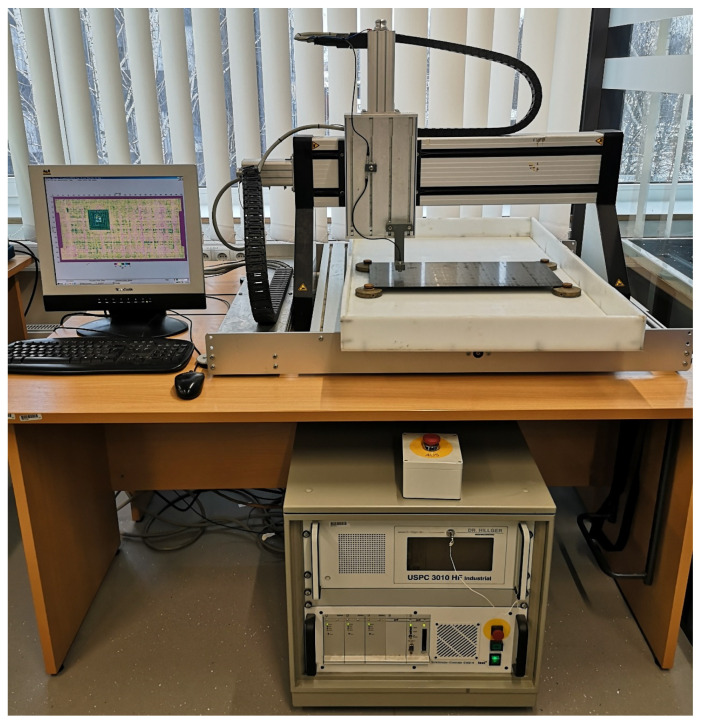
The ultrasonic experimental setup.
